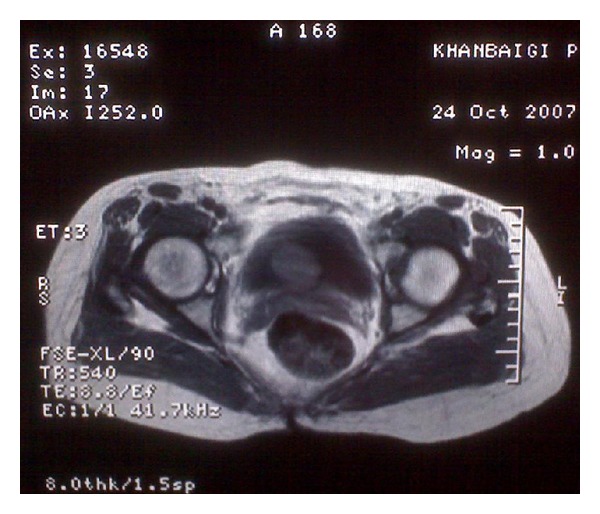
A well-defined 3 × 4 centimeter intramural mass was shown in MRI.
